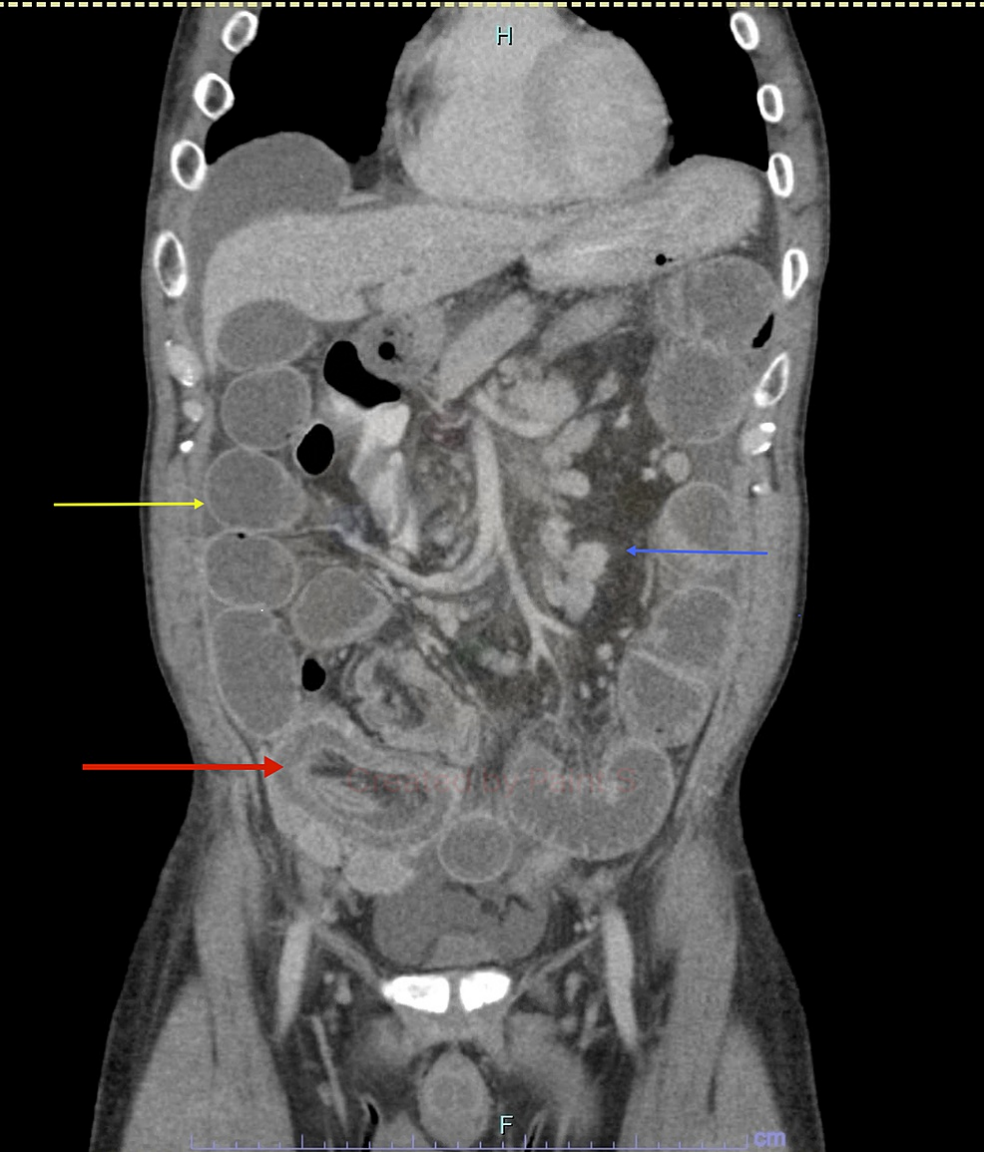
Abdominal CT scan with contrast (coronal view) revealing a distended small bowel (yellow arrow), mesenteric lymphadenopathy (blue arrows), and intussusception of distal ileum into cecum (red arrow).
